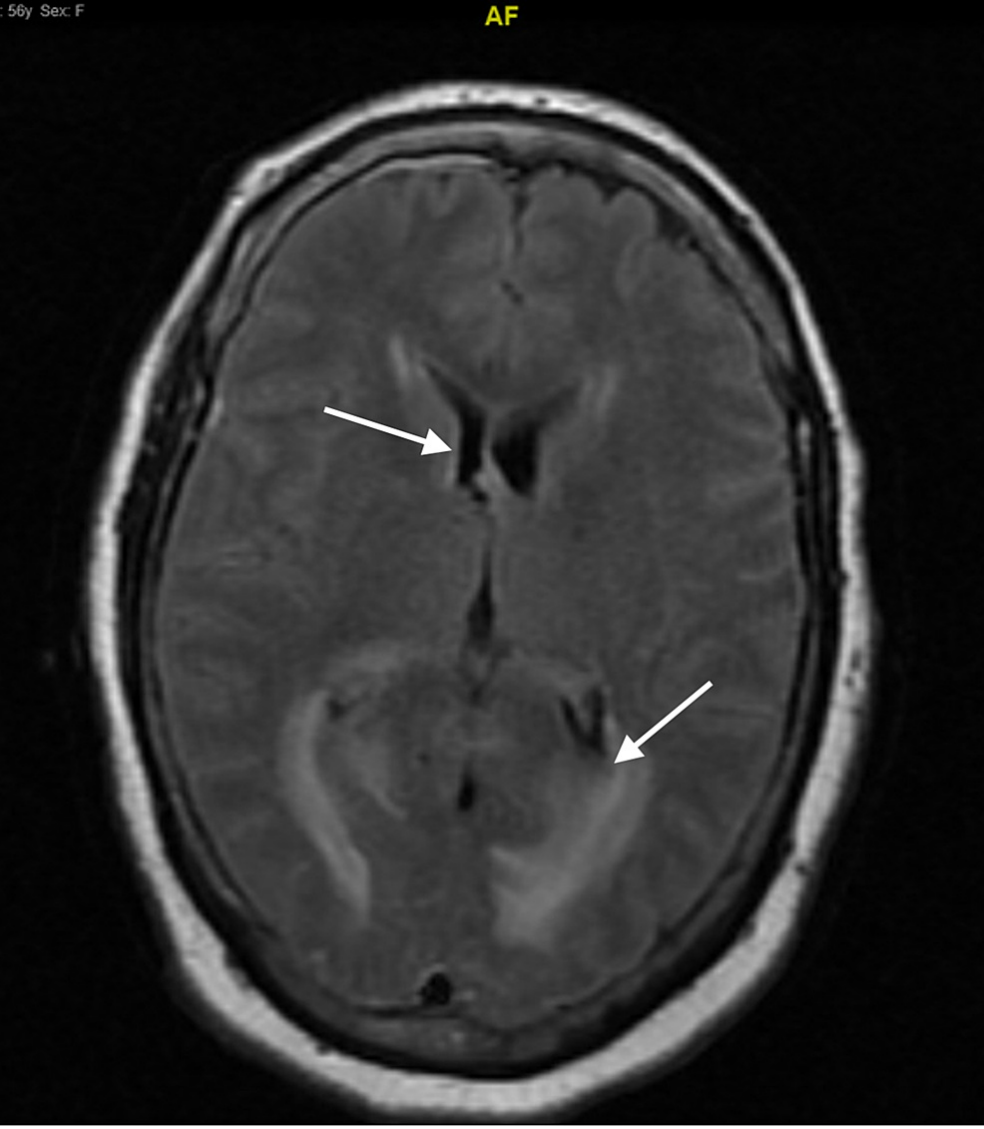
MRI of the brain without contrast in axial view on day 3 of hospitalization The image shows worsening diffuse brain edema with decreased ventricular size.
